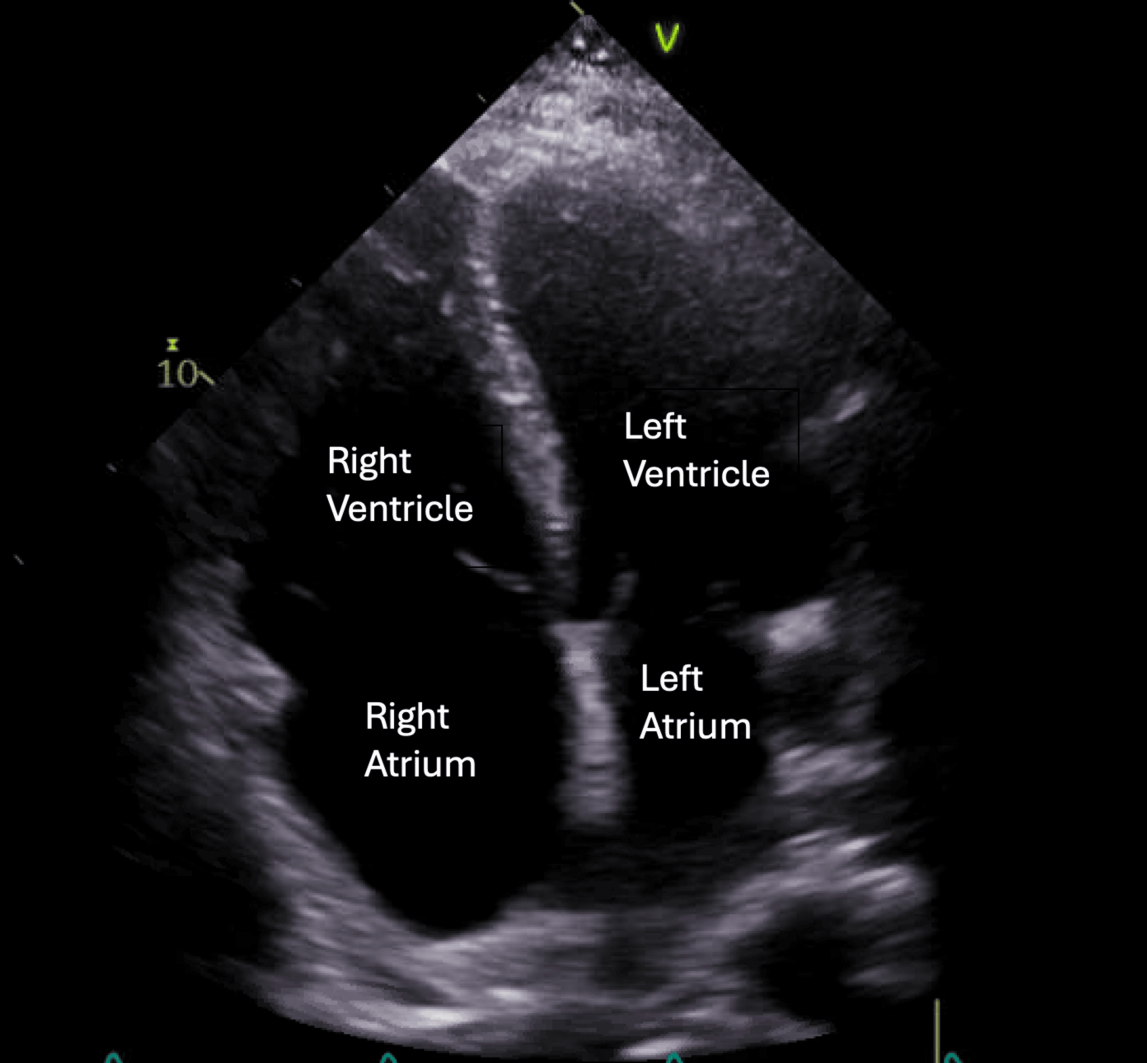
Transthoracic echocardiogram Apical four-chamber view revealed dilation of the right ventricle and right atrium, consistent with right-sided heart dysfunction. The septal motion abnormality observed is indicative of right ventricular pressure overload.
